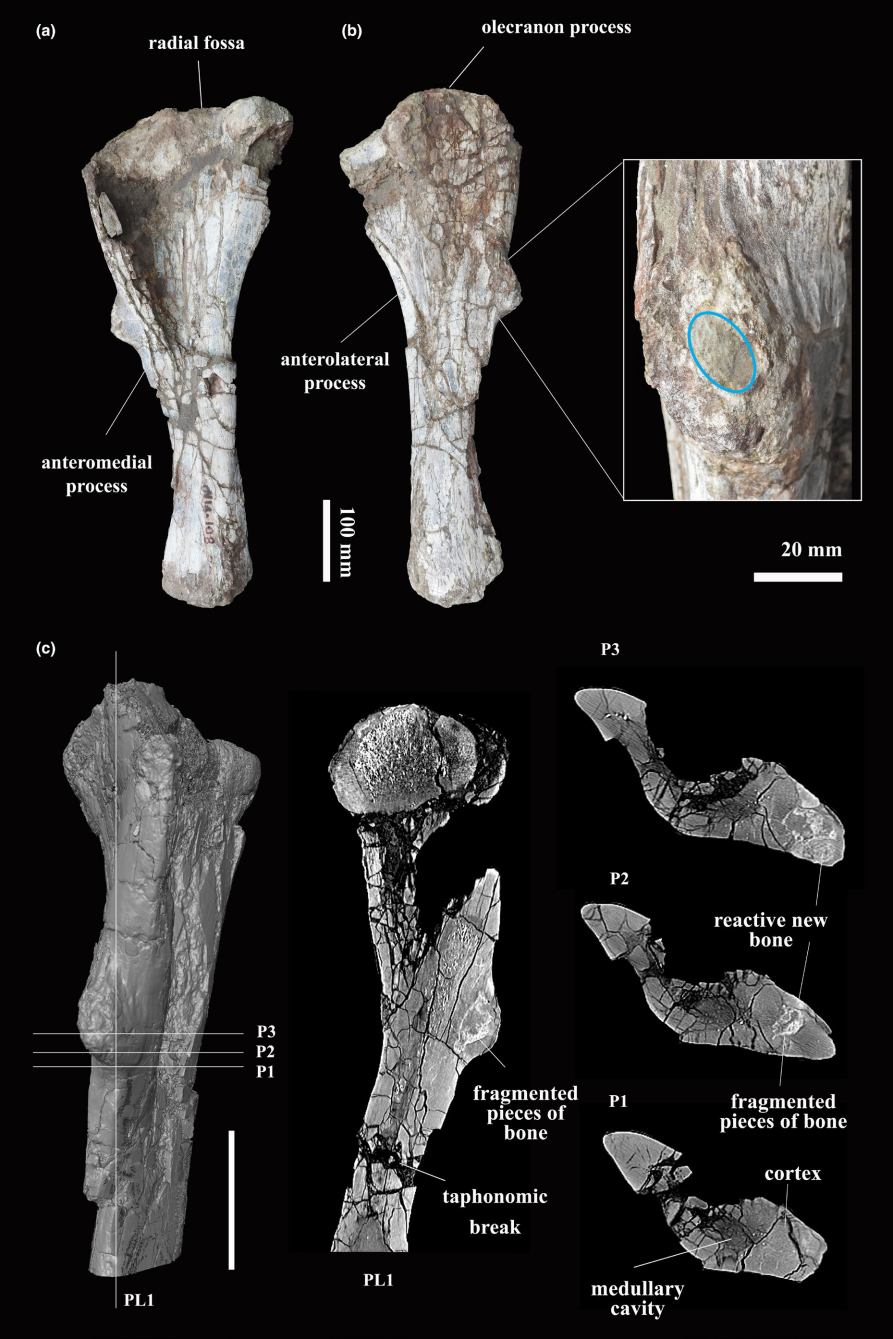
Mamenchisaurid ulna PN14‐108. (a) Gross external morphology of the ulna in lateral view. (b) Gross external morphology of the ulna in medial view. The box shows close‐up images of the external area that connects intracortically. (c) A 3D reconstruction in posterior view based on CT data and virtual thin sections showing the pathologic bone. PL1 indicates a sagittal view, whereas P1–P3 indicates cross‐sectional views. Scale bar equals 100 mm.
